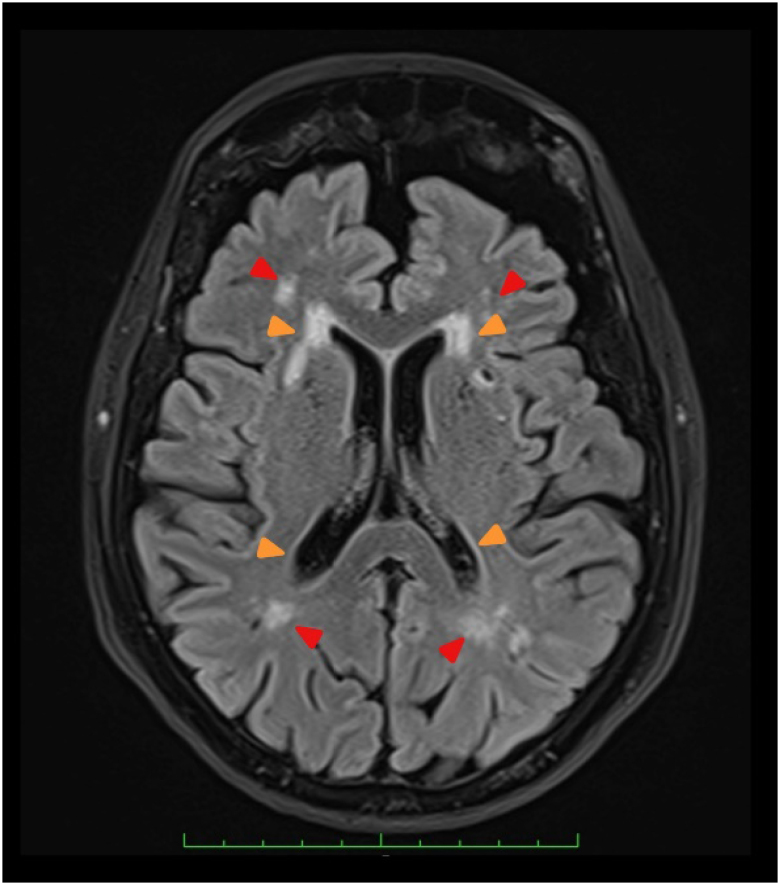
An 81-year-old woman with cognitive impairment. Brain magnetic resonance imaging in fluid-attenuated inversion recovery (FLAIR) sequence (axial view) showing subcortical (red arrowhead) and periventricular (orange arrowhead) white matter hyperintensities consistent with small vessel disease. Global brain atrophy is also observed (personal communication).
